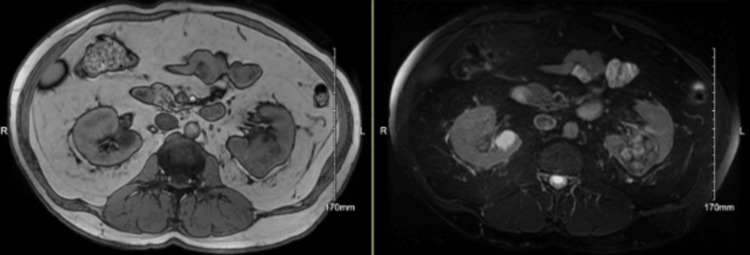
Axial slices from MRI of abdomen highlighting the multilobar renal mass in the left kidney. The left figure is a spoiled gradient recalled acquisition (SPGR) while the figure in the right is T2-weighted fat suppressed (T2FS).
